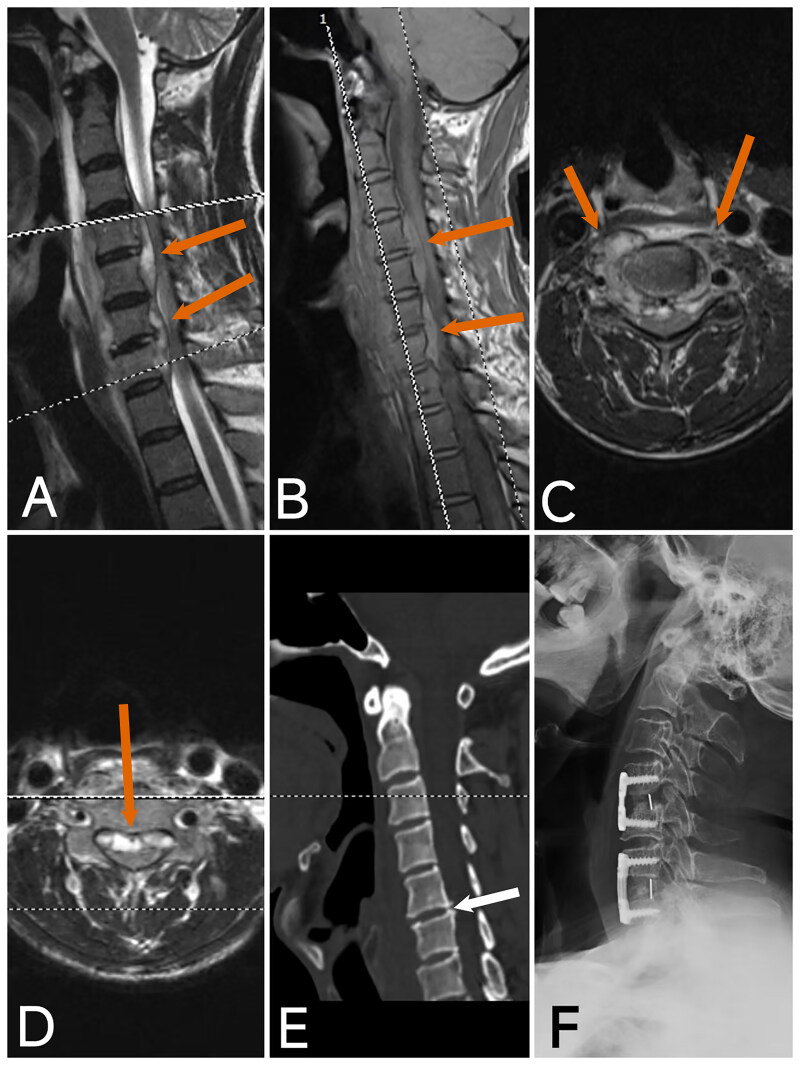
Image of case 1. A: T2-weighted imaging (T2WI); B: T1-weighted imaging (T1WI); shows a posterior epidural abscess in the spinal canal from C4 to C7, causing significant compression of the spinal cord (red arrow). C, D: Axial T2WI demonstrates epidural abscesses at two segments with notable compression of the spinal cord (red arrow). E: CT reveals cervical instability, loss of cervical curvature, loss of intervertebral disc height, and slight anterior displacement of C7 (white arrow). F: Postoperative X-ray at 5 months shows good positioning of the internal fixation, restoration of intervertebral height, recovery of cervical curvature, and intervertebral bone graft fusion.
